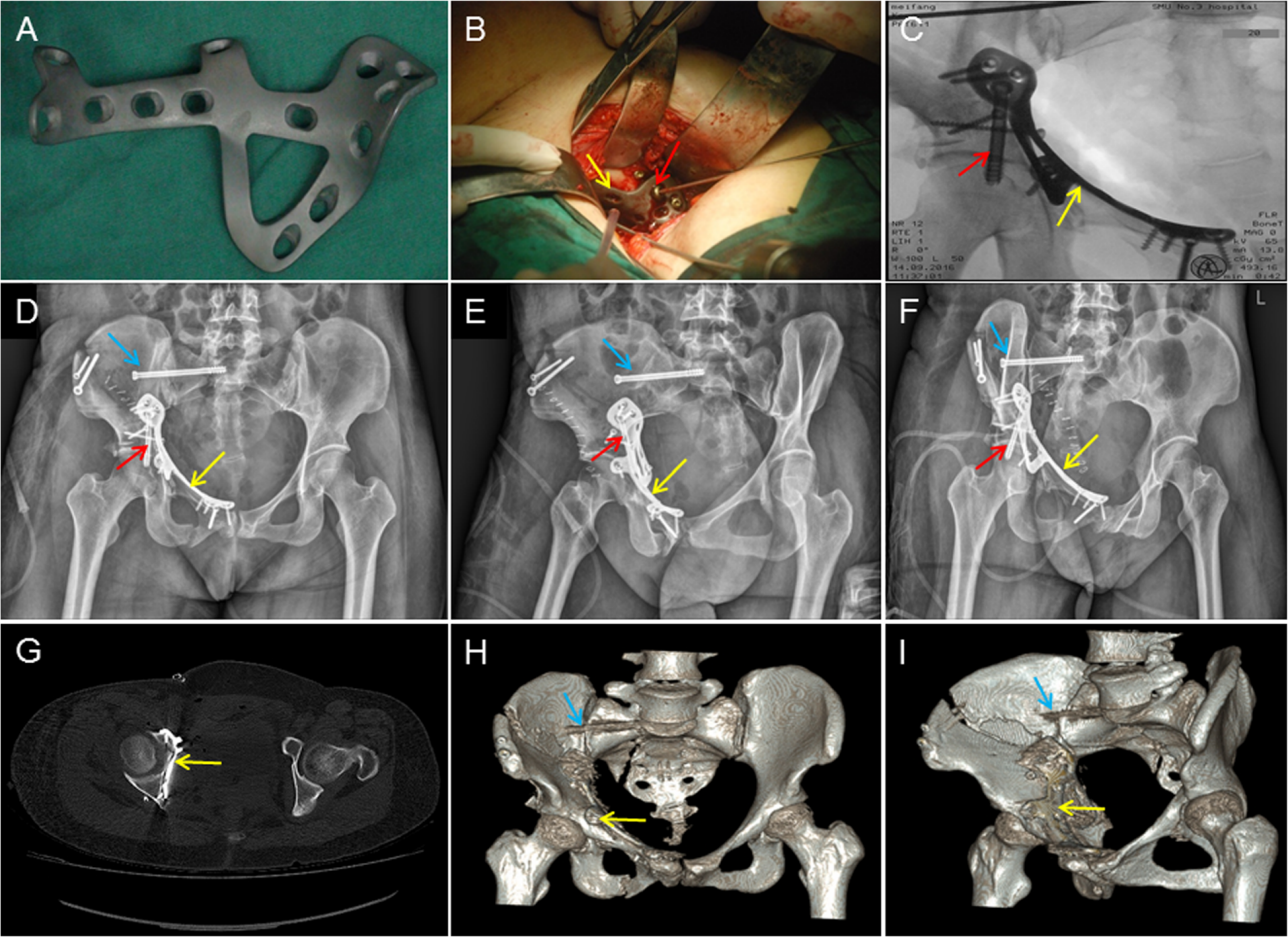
A 45-year old female who fell from a height and sustained a both-column fracture with QLP involvement, was treated with the 3DPPS plate. a 3DPPS plate with anodic coating; b Intra-operative fixation with the 3DPPS plate, yellow arrow is the 3DPPS plate, red arrow is the 6.5 mm lag screw; c Intra-operative radiographic data shows good reduction and fixation with the 3DPPS plate. Blue arrow is the percutaneous iliosacral screw; d–e Postoperative AP and Judet oblique view; f Axial images from a postoperative CT scan, demonstrating a near-anatomical adaptation of the 3DPPS plate; h–i AP view and Judet view at 3-month follow-up
